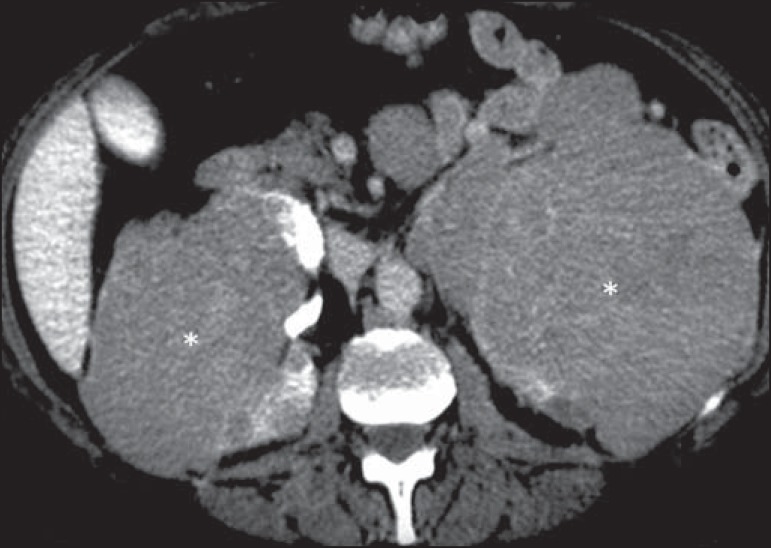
Primary renal lymphoma. Contrast-enhanced axial CT of the abdomen showing
